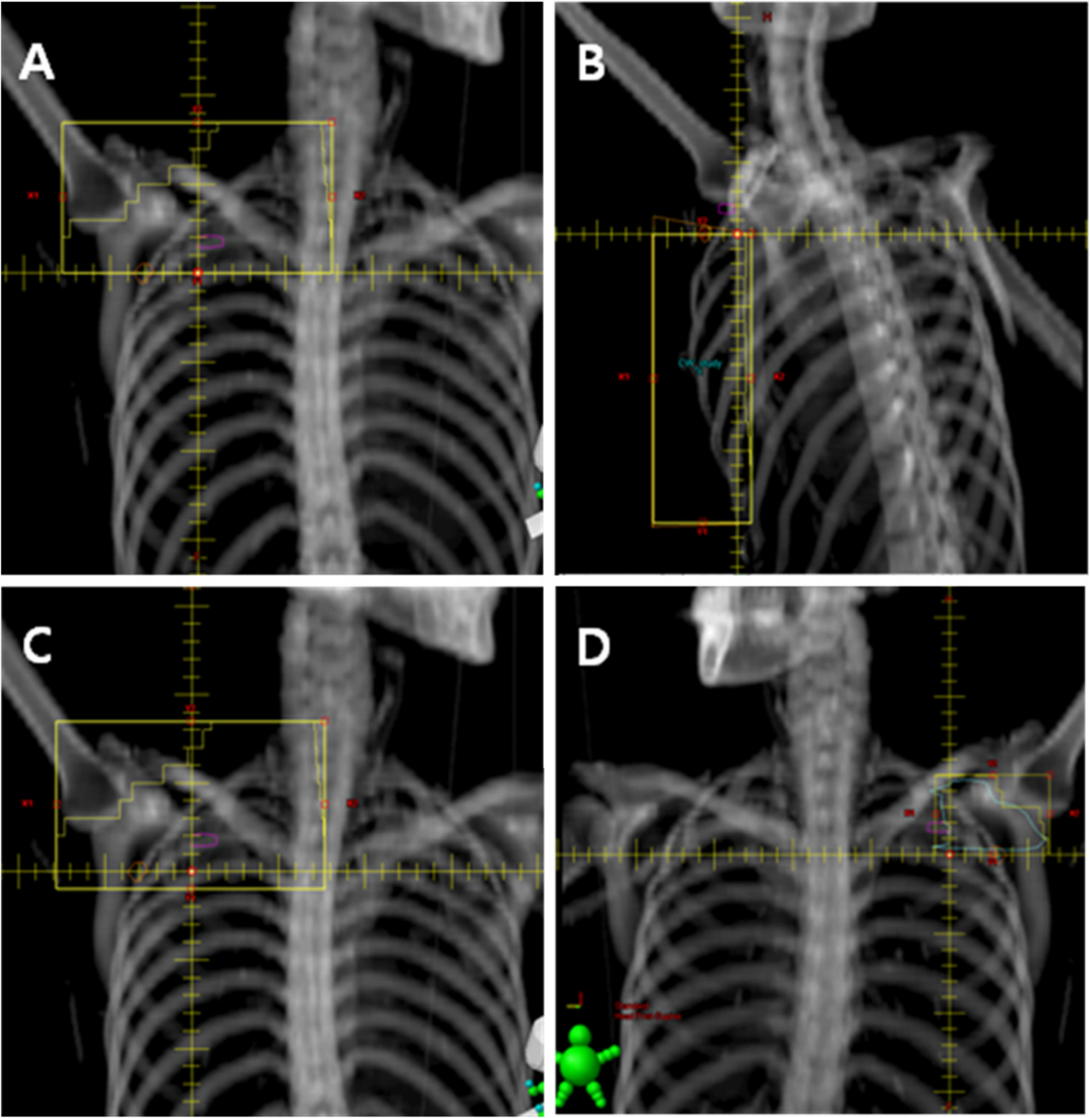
Digitally reconstructed radiographs (DRR) image of each field. A: Anterior field. It is the historically designed field. B: Tangential fields. They also follow the historically designed fields. C: Modified anterior field. It is 1 cm larger below the junction compared to the historical field. D: Posterior axillary boost beam. Sky blue line represents the area received the dose below 90% of the prescribed dose.
